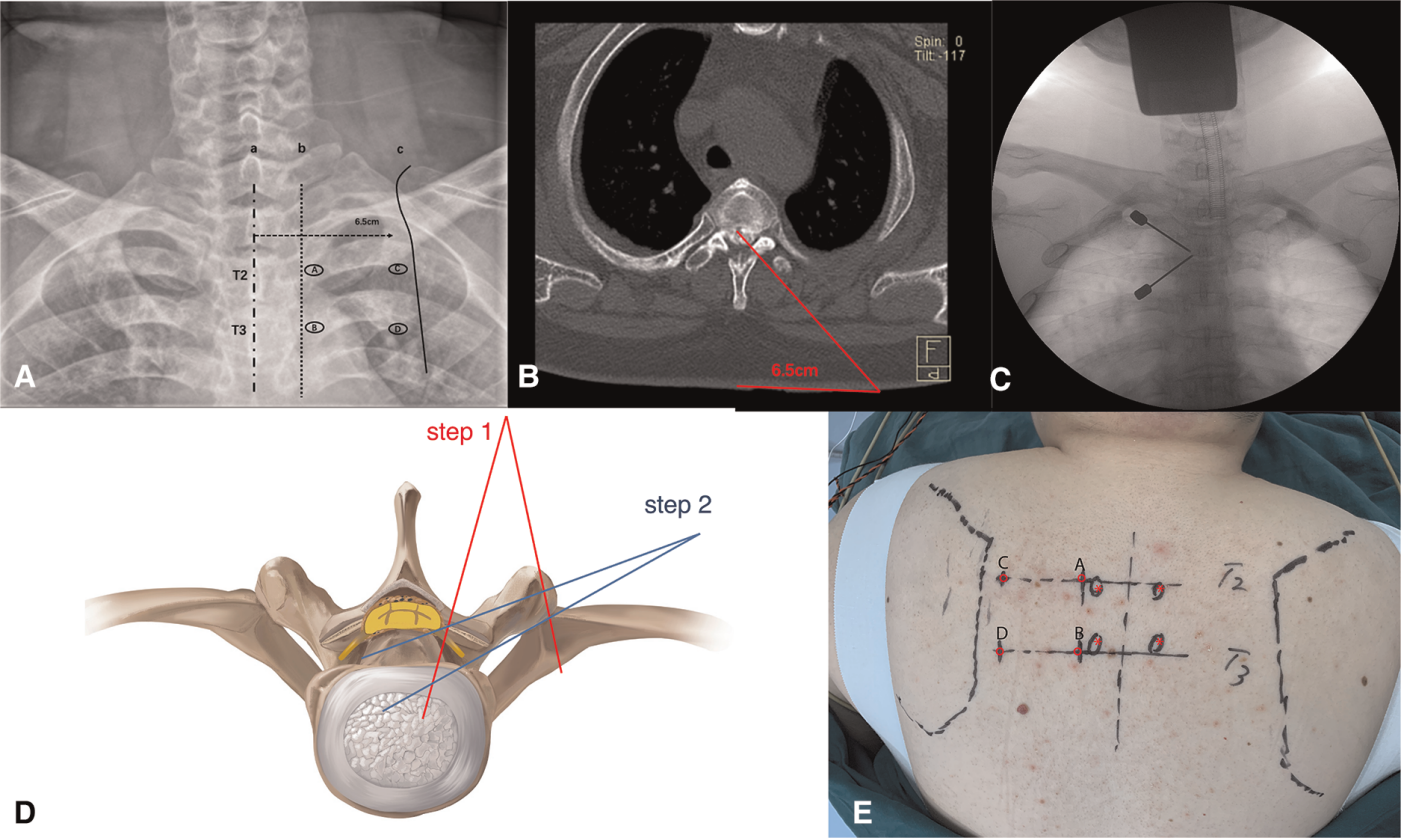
(A,E) The localization of the portals on the preoperative anteroposterior radiograph and the body surface. (B,D) Preoperative assessment of the extent of spinal cord decompression. (C) Puncture at portals A and B, and the target is the left T2–T3 facet joint.
